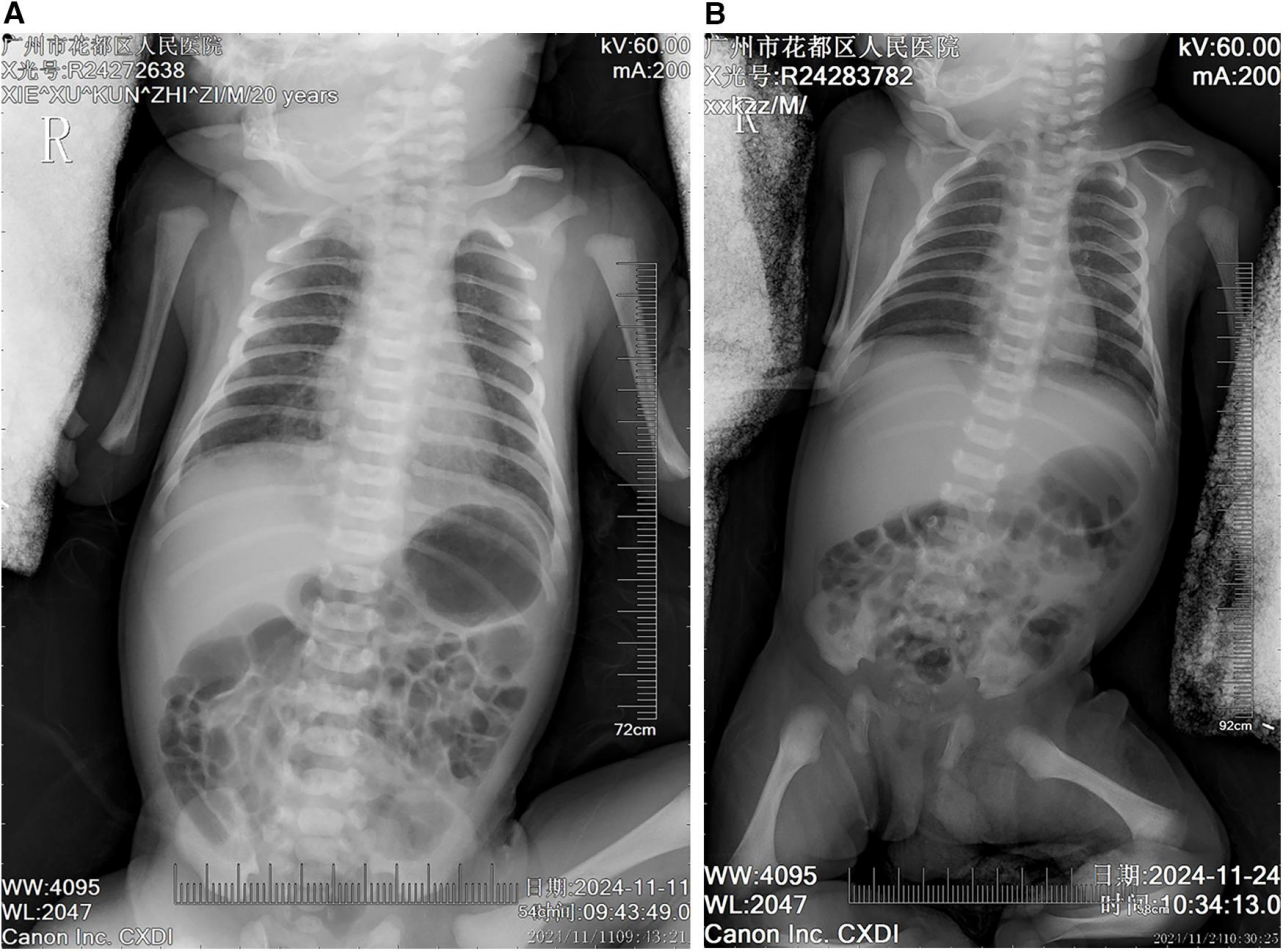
Chest radiographic changes in a neonate with meconium aspiration syndrome before and after treatment (A) initial chest radiograph (day 0 of life) showing diffuse bilateral pulmonary infiltrates with hyperinflation, patchy opacities, and flattened diaphragm consistent with meconium aspiration syndrome. Note the increased lung markings and coarse reticular pattern throughout both lung fields. (B) Follow-up chest radiograph (day 14 of treatment) demonstrating significant improvement in pulmonary aeration with resolution of the diffuse infiltrates. The lung fields appear clearer with normal lung volumes and diaphragmatic contour, indicating successful response to respiratory support and antimicrobial therapy.
